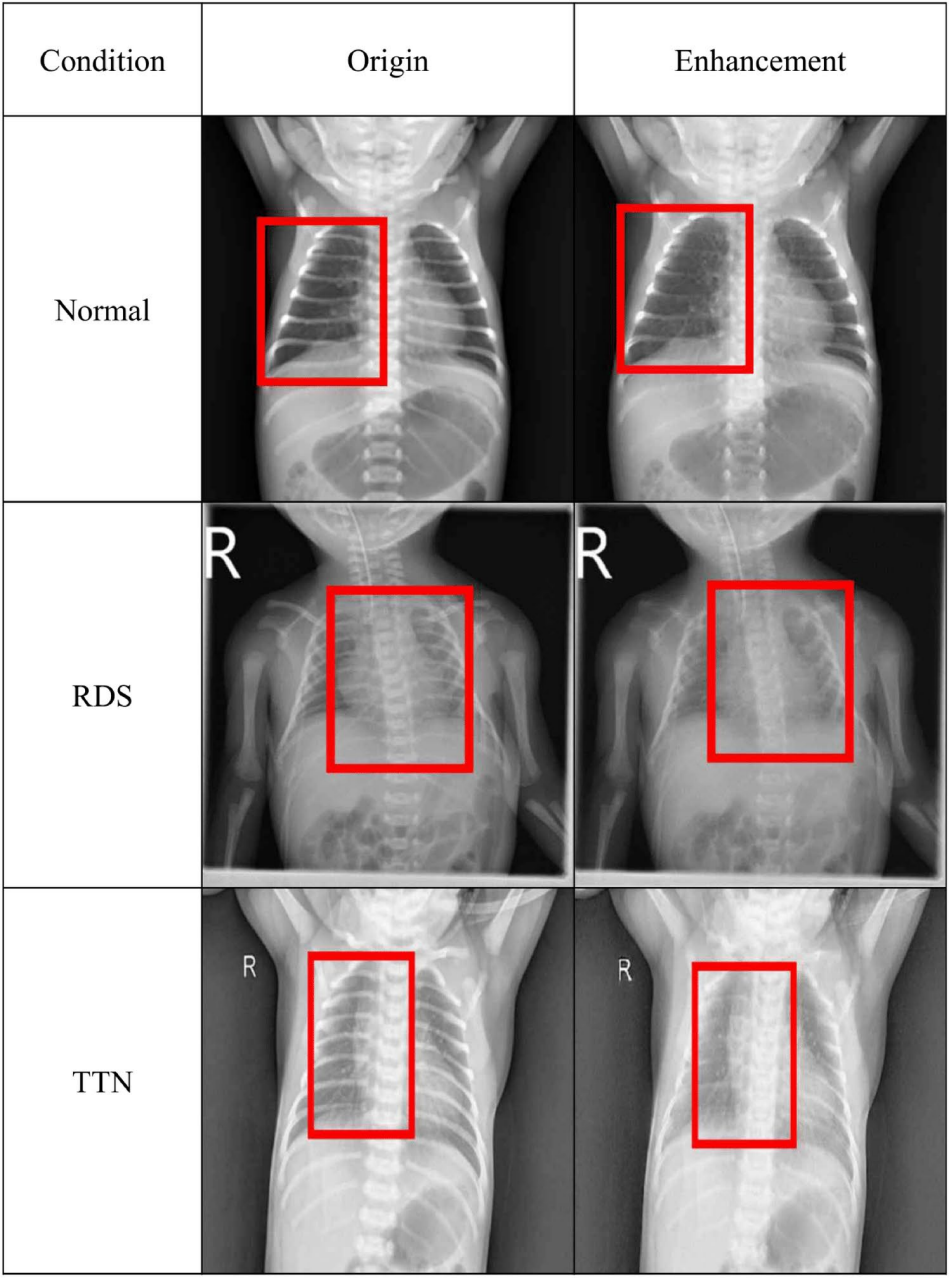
Comparison of original and enhanced X-ray images after bone removal for different conditions.
